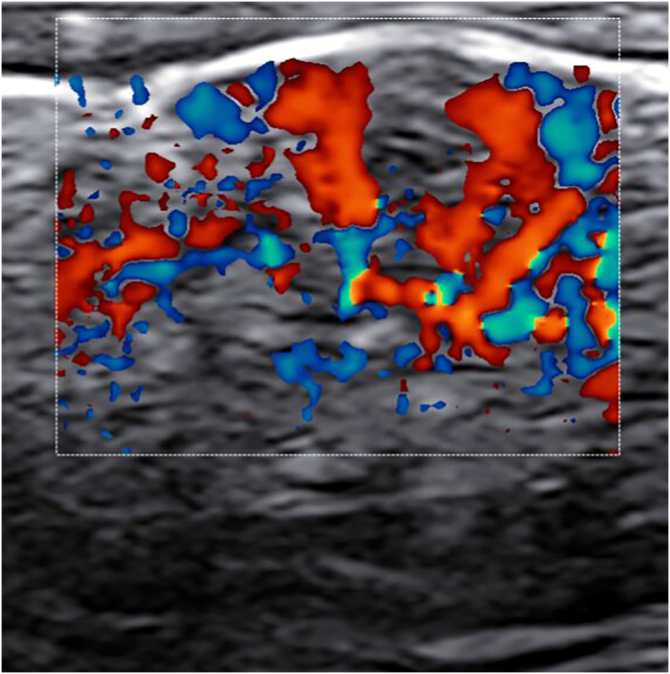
Ultrasound findings (22 Hz). Color-Doppler of a pseudopustule that highlights the marked intralesional vascularization, together with dermo-epidermal thickening and focal dermo-hypodermal hypoechogenicity.
